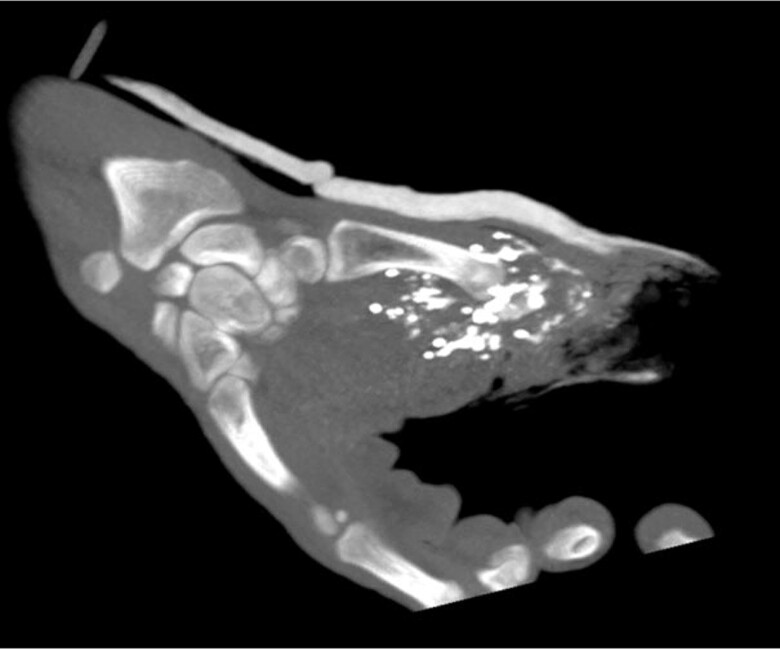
Computed tomography coronal images demonstrating multiple shrapnel in the right hand.
